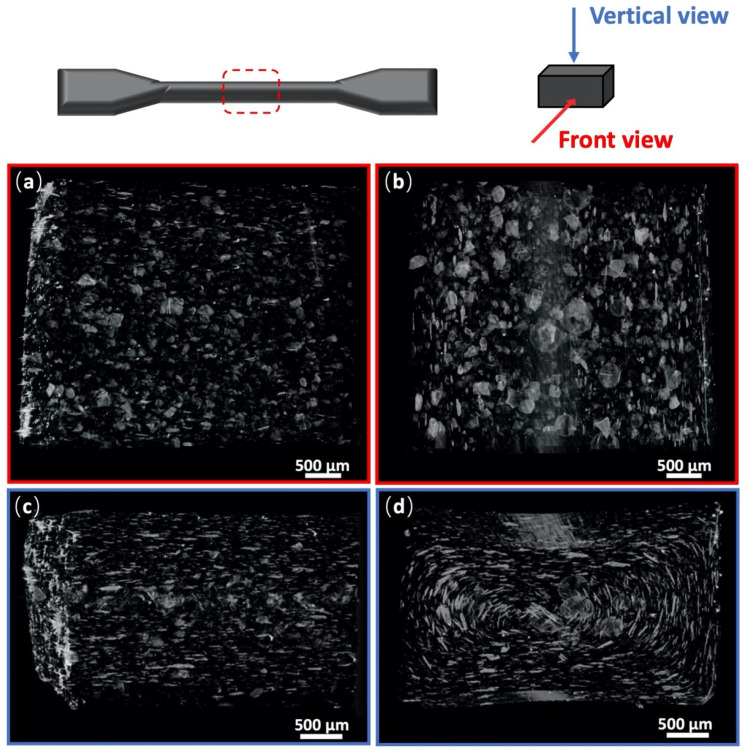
Micro X-ray CT images of: (a) front view and (c) vertical view of BMM-2.5 wt% GNPs; (b) front view; and (d) vertical view of BM-2.5 wt% GNPs.
